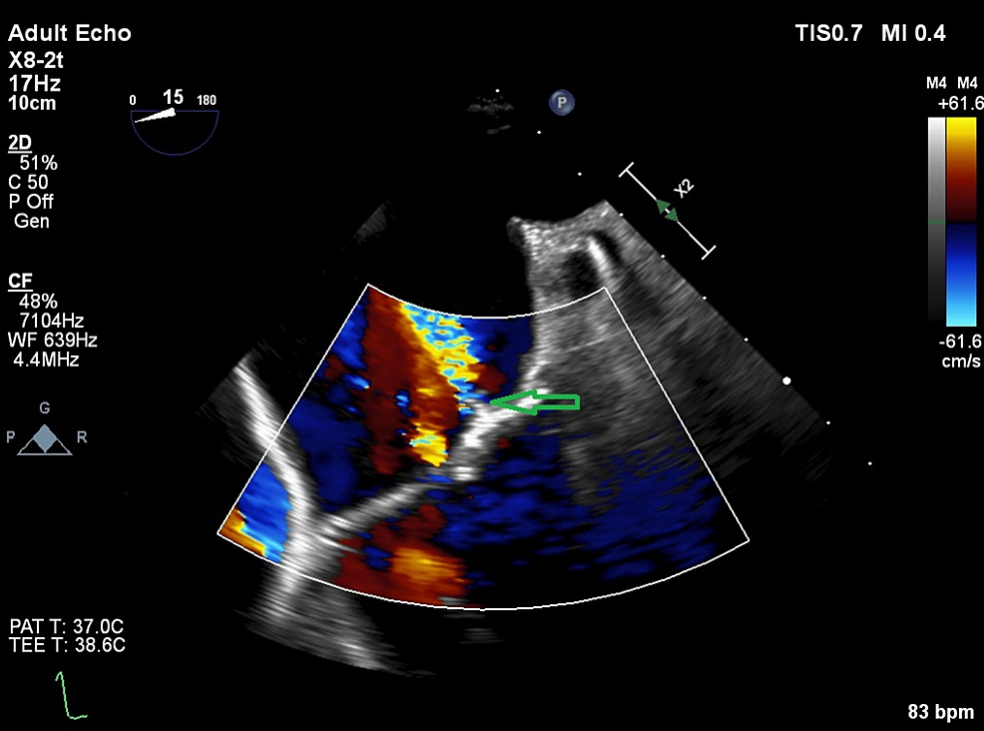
A transesophageal echocardiography of the patient with a perforation of the mitral valve (arrow).
